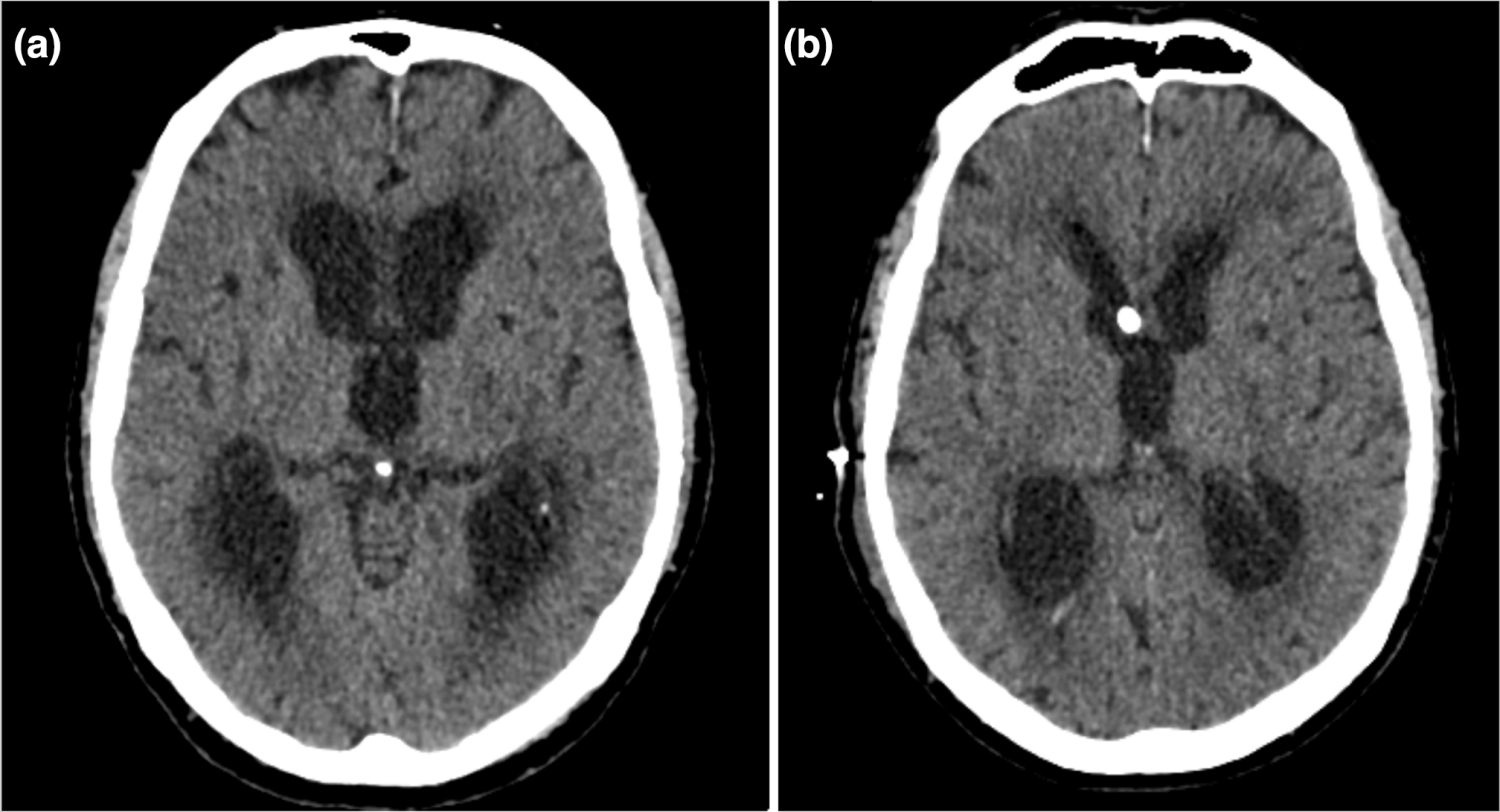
Axial non-contrast computed tomography scans taken (a) 8 weeks post-operatively, showing hydrocephalus, and (b) following insertion of an external ventricular drain into the right lateral ventricle, enabling decompression of the ventricular system.
